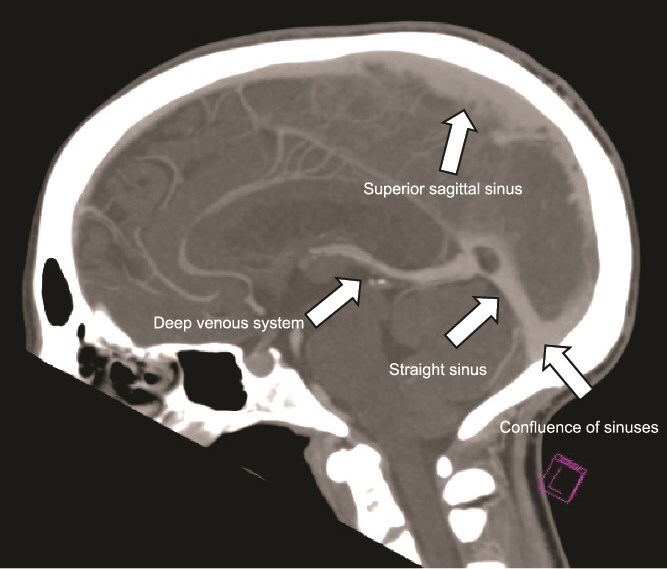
Normal CT Venogram demonstrates contrast within the significant venous sinuses: Sagittal sinus with cortical vein tributaries, transverse and straight sinus, and deep venous system circulation. ^*^(case courtesy of frank Gaillard, Radiopaedia.org, rID: 28100).
